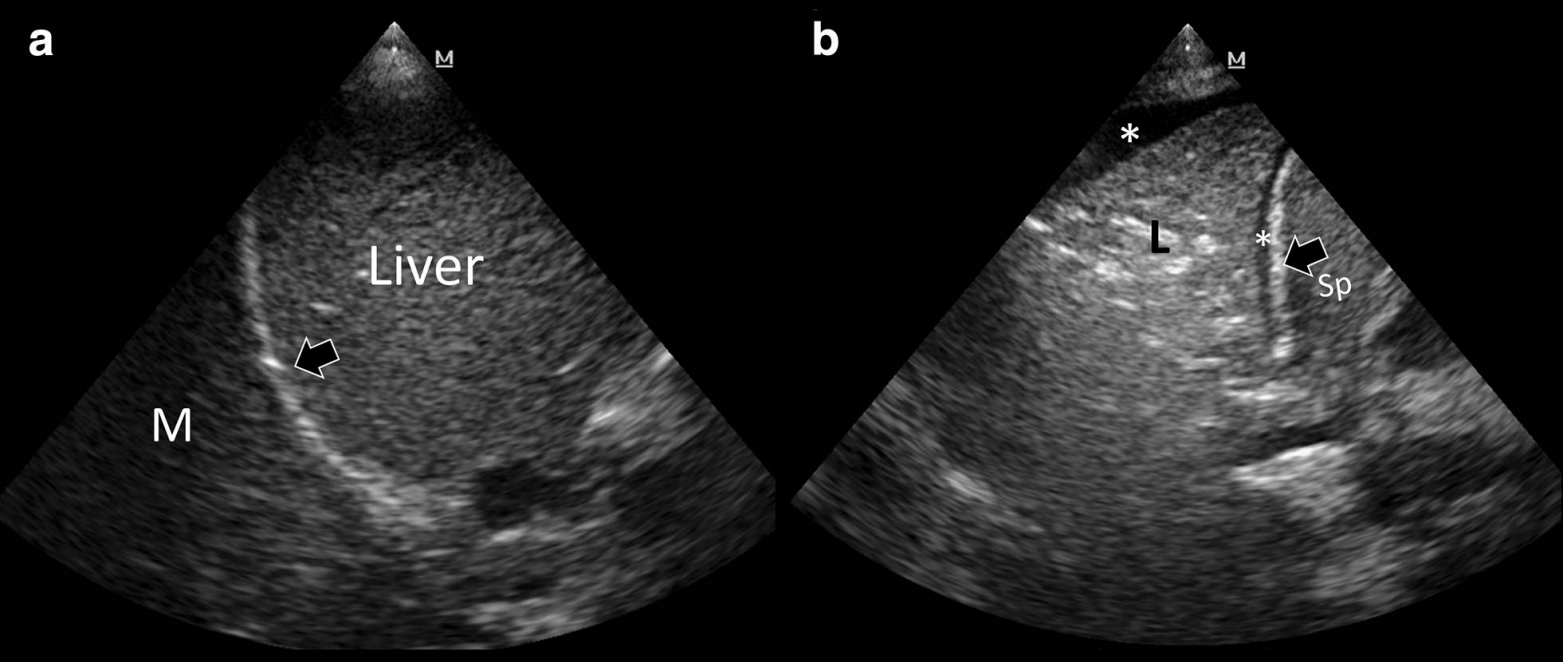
a Mirror image artifact (M), showing a similar tissue-like pattern in comparison with the liver; *arrow*: diaphragm. b Lung consolidation (L). Distinctively, it is shown a tissue-like pattern, sonographic air bronchogram and pleural fluid (*asterisks*); *arrow* diaphragm, *Sp* spleen
